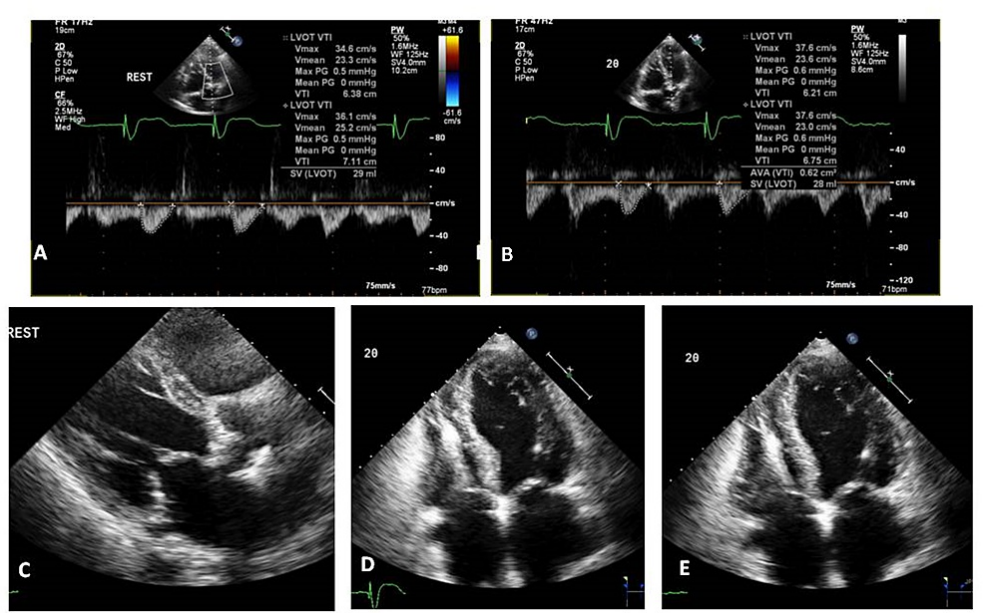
Dobutamine stress echocardiography shows failure to augment cardiac output at peak stress. There was no difference in cardiac output between baseline (panel A) and peak stress (panel B). The aortic valve was severely calcified (panel C). LVEF was severely reduced at peak stress (panel D: end systolic; panel E: end diastolic). LVEF, left ventricular ejection fraction
